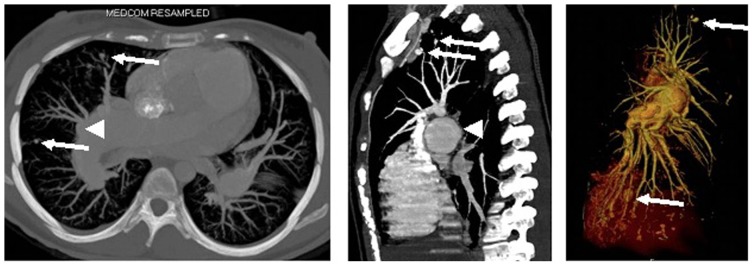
CT angiography from a 23 year old iPAH patient who died from massive hemoptysis. Panel A is a transverse section showing central pulmonary artery dilatation and small saccular aneurysms in more peripheral vessels (small arrows). Note the irregular borders of the central vessels. Panel B shows a coronal reconstruction with aneurysmatic changes to the main pulmonary artery (large arrow) and peripheral vessels (small arrows). Panel C is a 3 D reconstruction.
